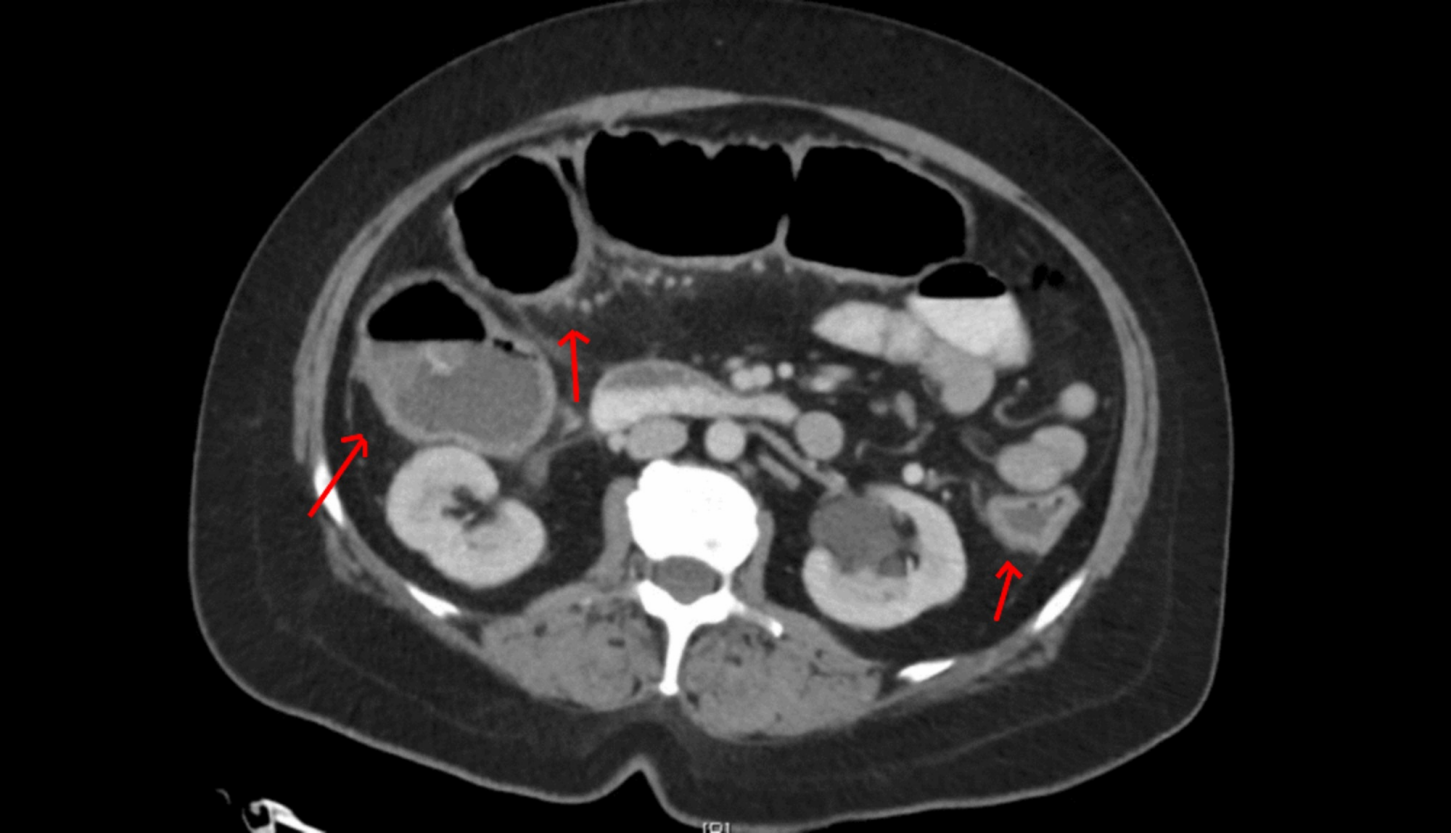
CT scan abdomen and pelvis Pancolitis
